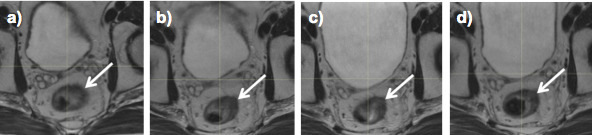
Changes in rectal tumour volume (arrow), as seen on weekly MRI during treatment at (a) week 0, (b) week 2, (c) week 3 and (d) week 4.
